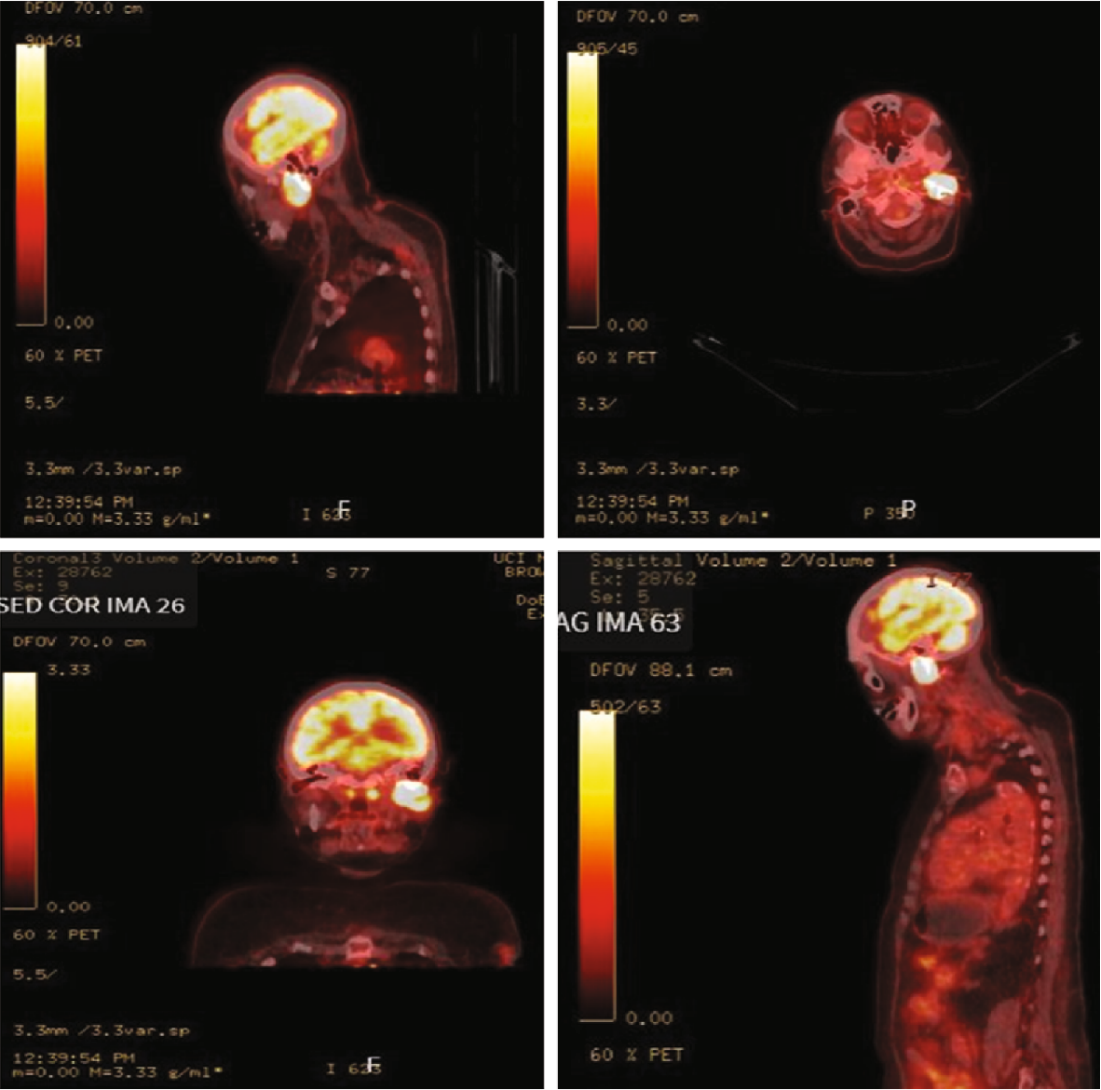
PET/CT image showed a hypermetabolic left parotid mass compatible with a known neoplasm involving both the superficial and deep parotid lobes, with activity measuring up to 12.0 SUV. No significant FDG-avid lymphadenopathy.
